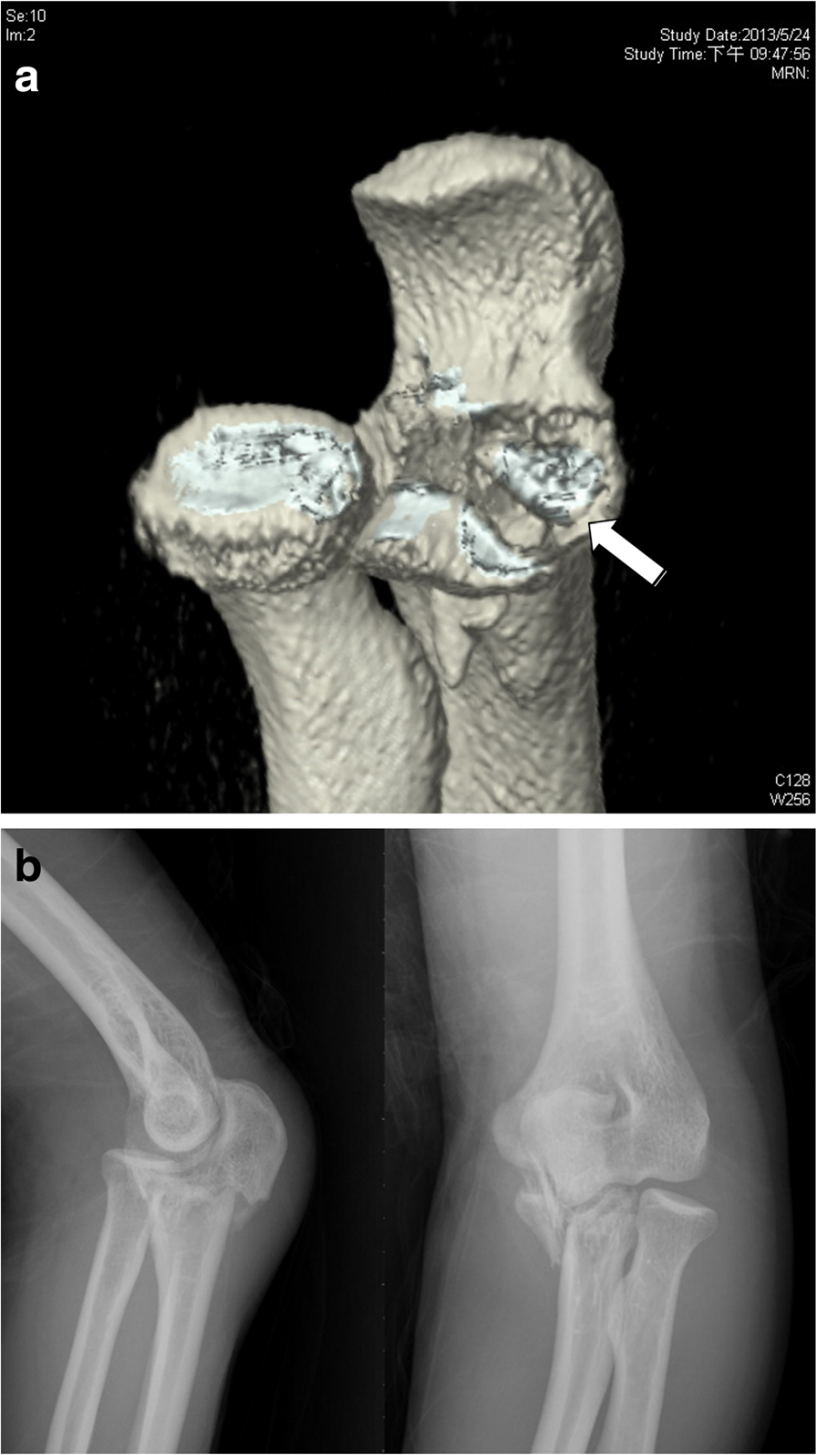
Two cases of anteromedial facet fractures. a Three-dimensional computed tomography scans showing an articular depression fracture (white arrow) close to the base of the coronoid process. b Plain radiographs showing an olecranon fracture with a displaced anteromedial facet fracture of the coronoid process
